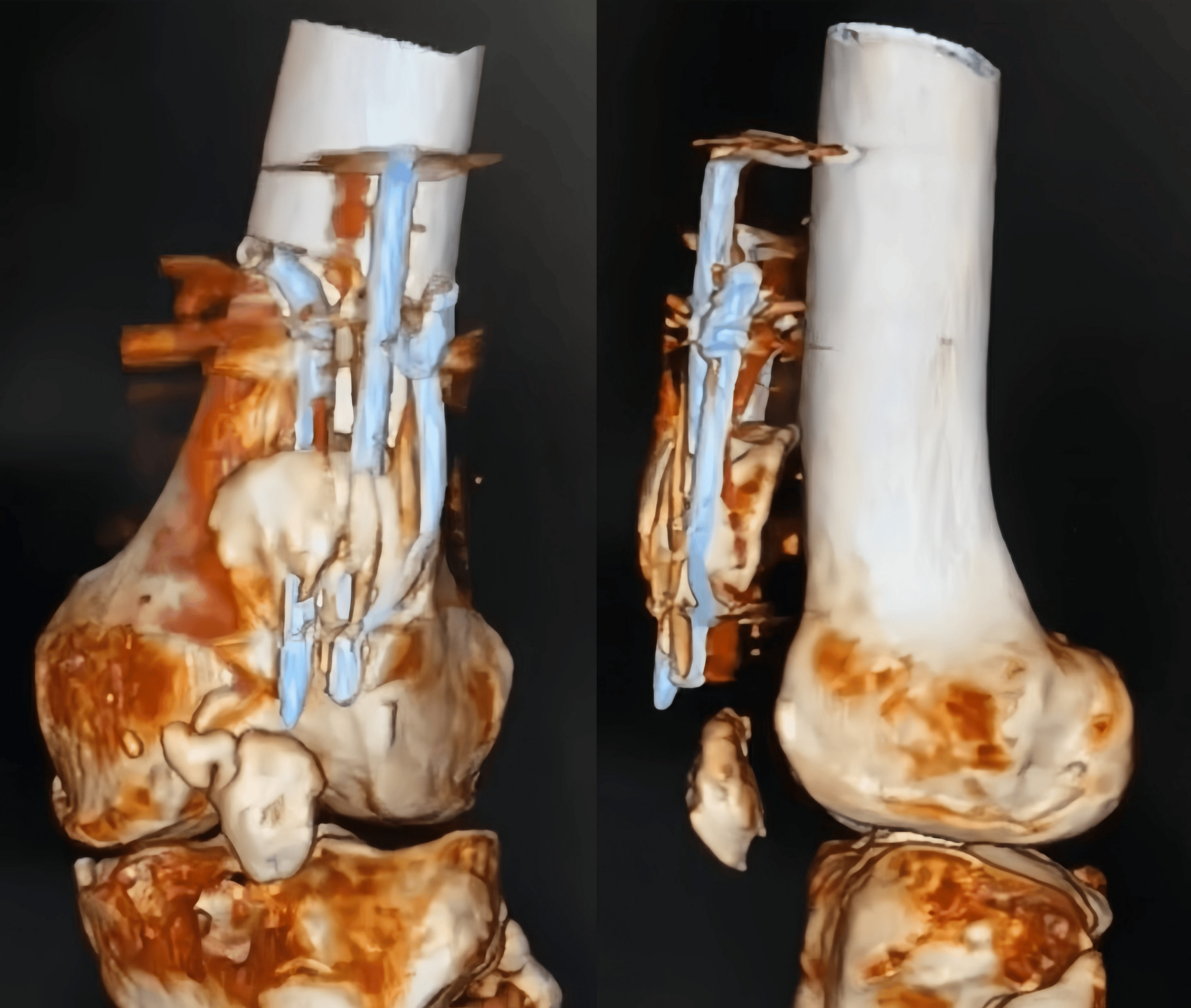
Computed tomography (CT) of the left knee joint. CT scan images showing comminuted and displaced fracture of patella leading to separation of upper and lower fragments of the patella with evident tension band wiring and K-wire fixation in the upper fragment of the patella
